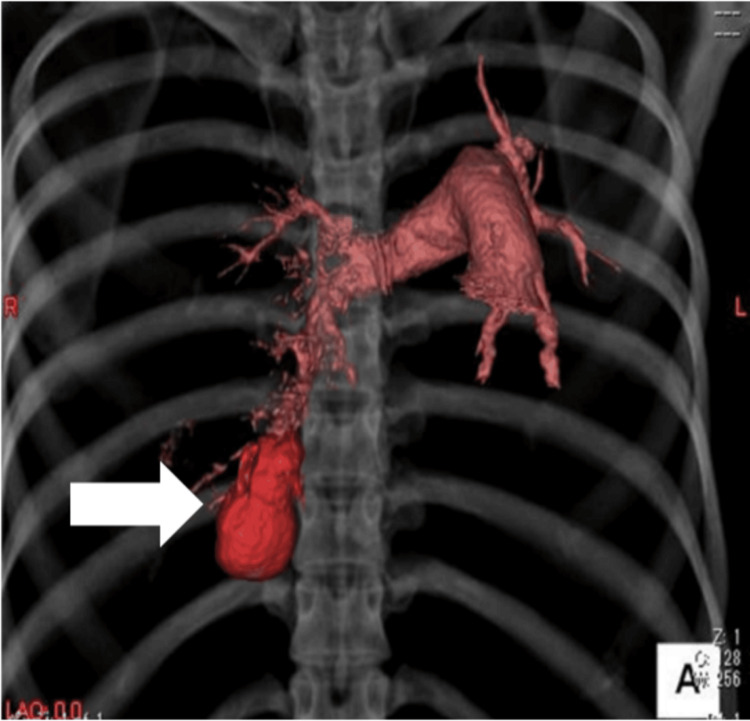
Contrast-enhanced chest computed tomography scan Contrast-enhanced chest computed tomography scan showing the pulmonary arteriovenous malformation (arrow).
